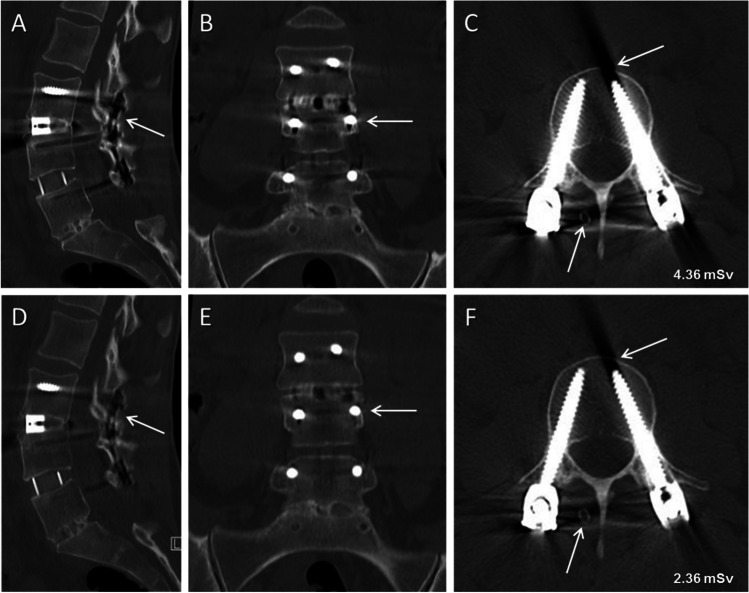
A 38-year-old female with lumbar instrumentation L3-5. Reformatted sagittal (A and D), coronal (B and E) and axial (C and F) CT images of the 120 kV standard CT (A-C) and of the 140 kV low-dose CT with tin filtration (D-F). Both datasets show clear depiction of anatomy and weak image noise. The tin-filtered low-dose CT demonstrates significantly less image artifacts (arrows) compared to the standard CT
